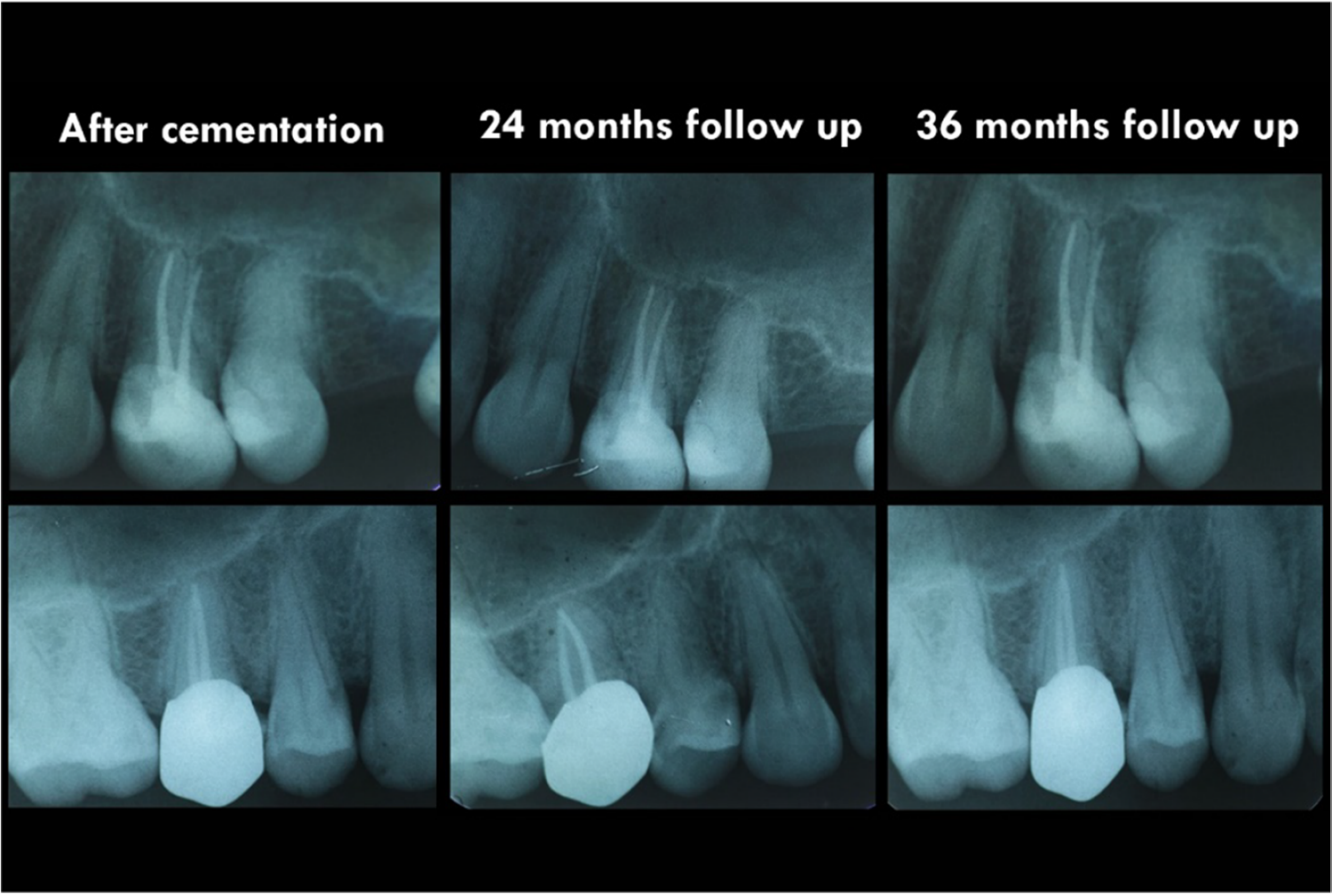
Radiographic images: showing Celtra Duo (above) and KATANA (below) crowns during follow-up period. No pathology, no pockets, and there was a harmonious transition between crowns and neighboring teeth
